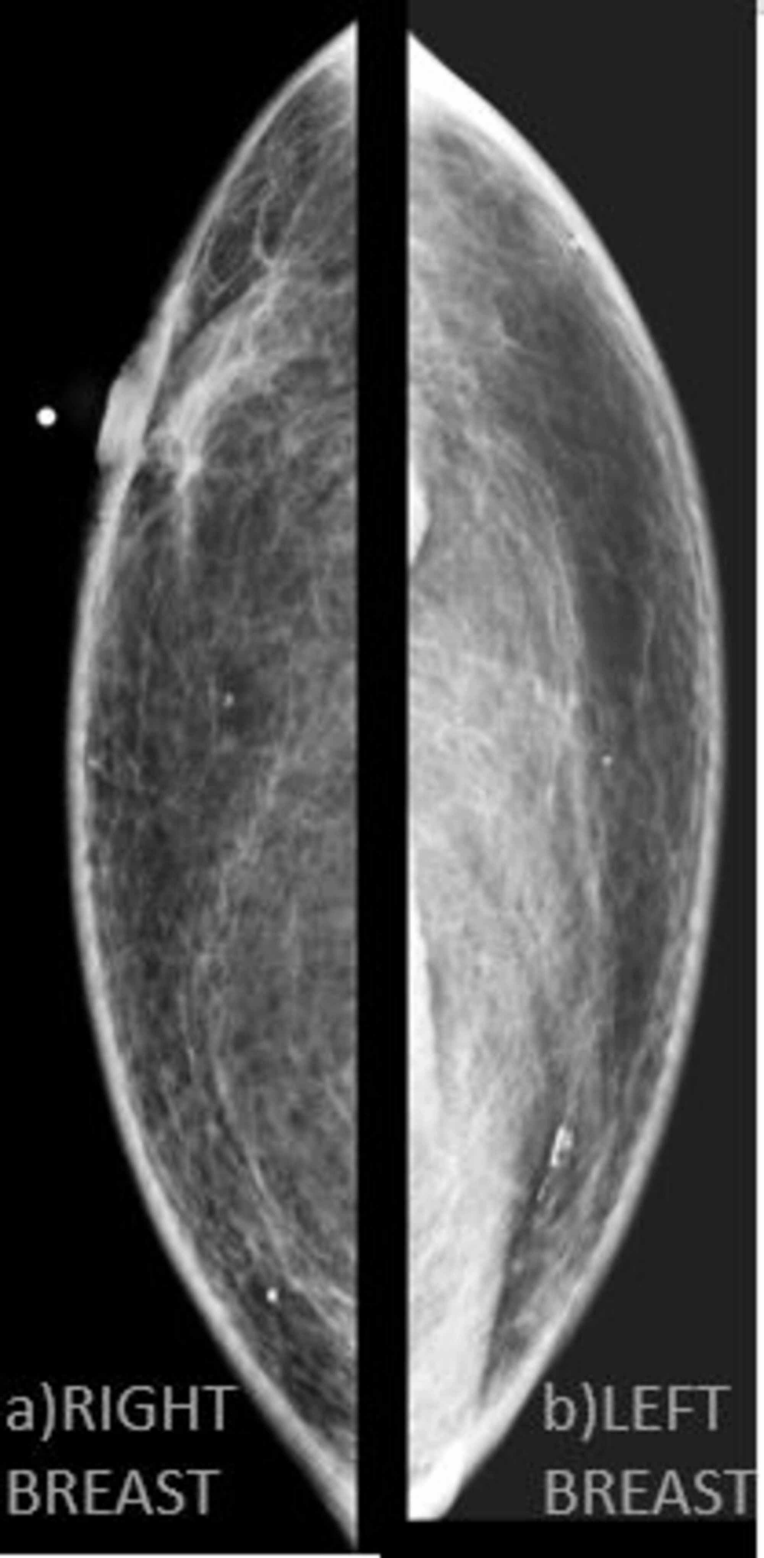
Mammography of the patient Mammography of the patient's breasts was not able to locate the mass; therefore ultrasound was needed to further understand our patient's condition. Right breast and left breast are denoted by grey text
